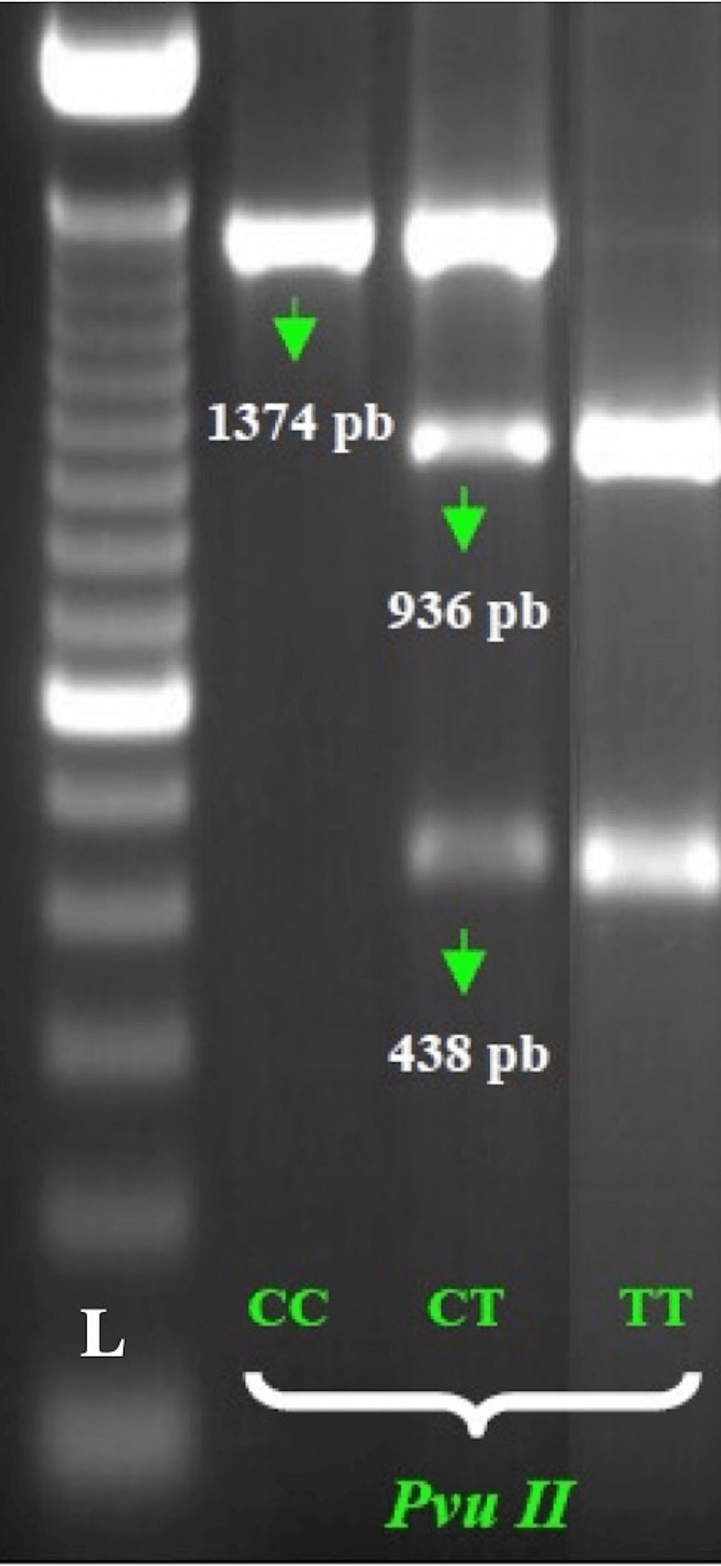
ESR1 rs2234693 genotypes The CC genotype is represented by one band of 1374 bp, the CT genotype is identified by three bands of 1374 bp, 936 bp, and 438 bp, and the TT genotype is represented by two bands of 936 bp and 438 bp. ESR1: estrogen receptor 1.
